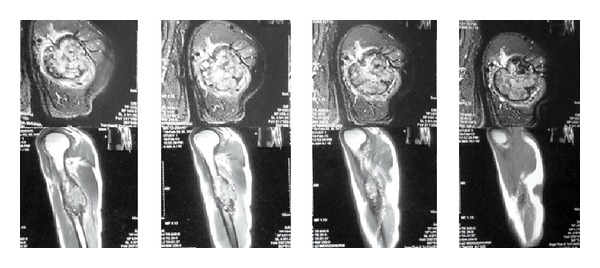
MRI of left upper extremity showing osteolytic lesion in left humerus with marrow edema and soft tissue infiltration.
